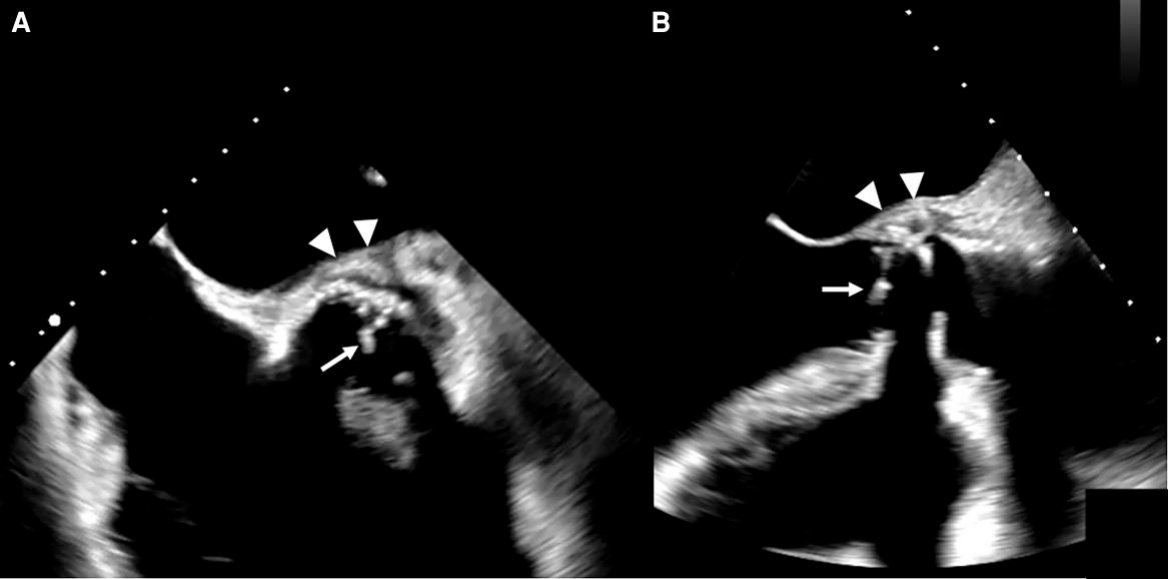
Transoesophageal echocardiography: transoesophageal echocardiography shows ring abscess [(A) short-axis view and (B) mid-oesophageal long-axis view, arrow heads] and a mobile vegetation on the mechanical aortic valve prosthesis prolapsing into the left ventricular outflow tract [(A and B), arrows].
